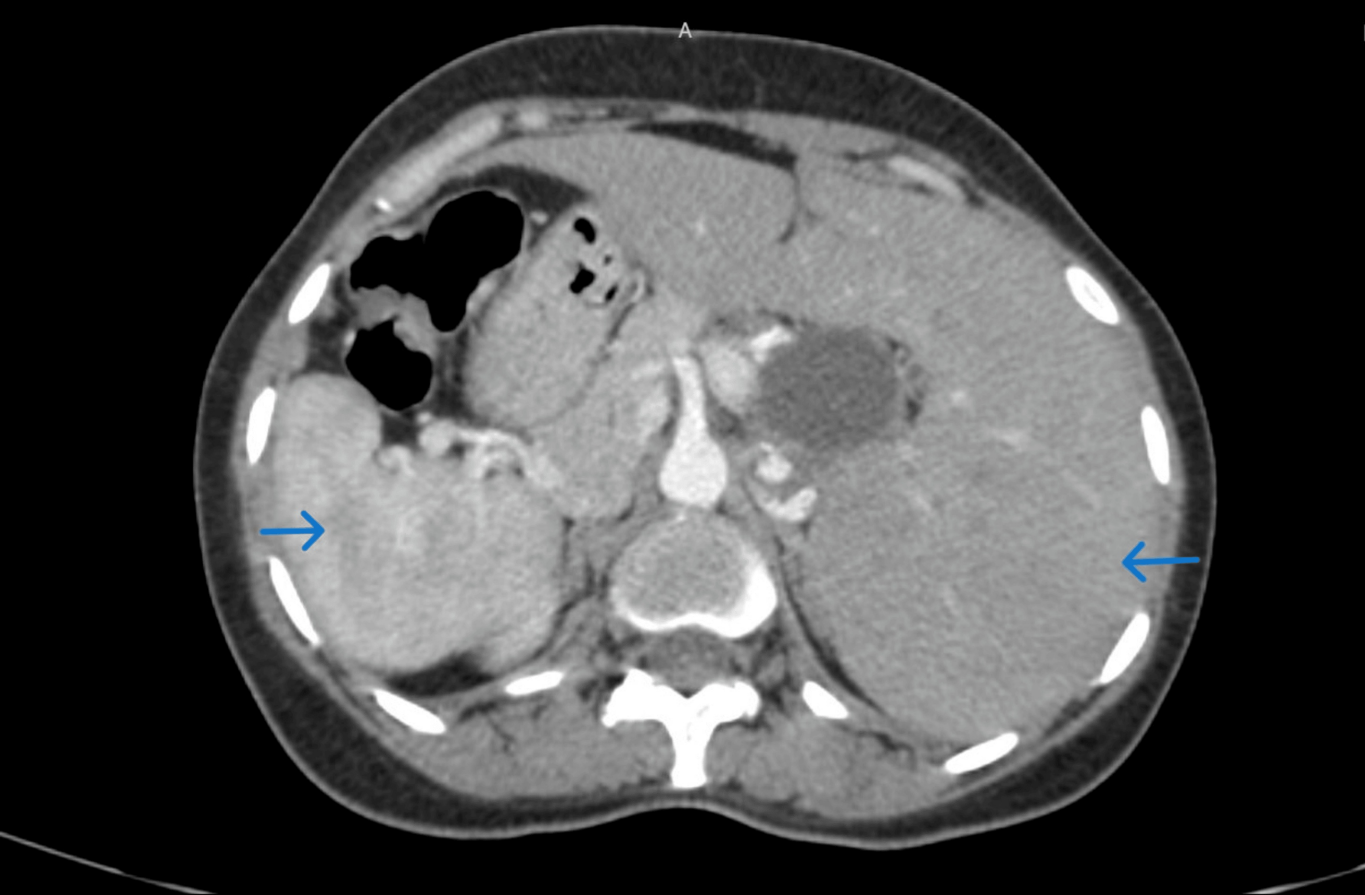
Axial late arterial phase contrast CT scan image of the abdomen shows the spleen and stomach on the right and liver located on the left side of the abdomen. The choledochal cyst was also visualized. CT: computed tomography
